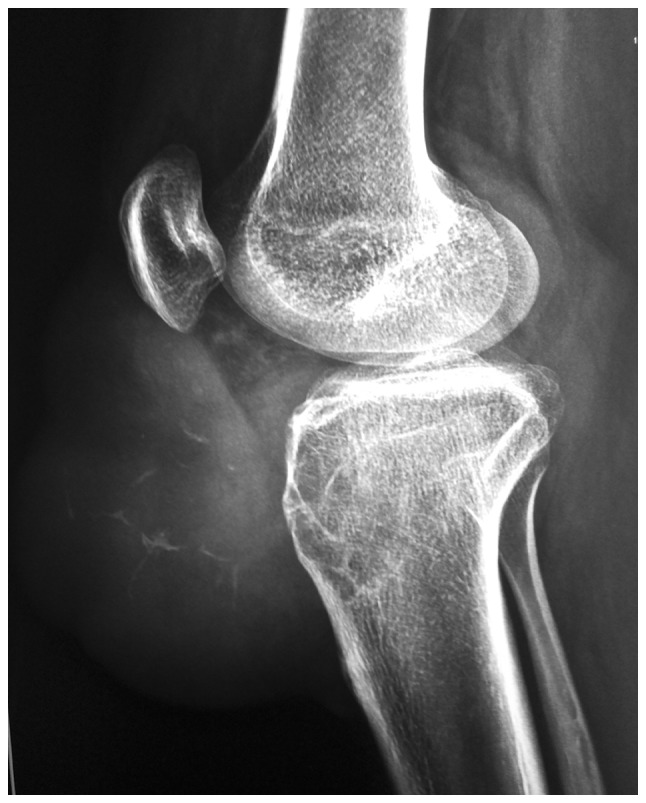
Lateral view X-ray showing a lytic area in the proximal end of the tibia presenting with soft tissue calcification.
